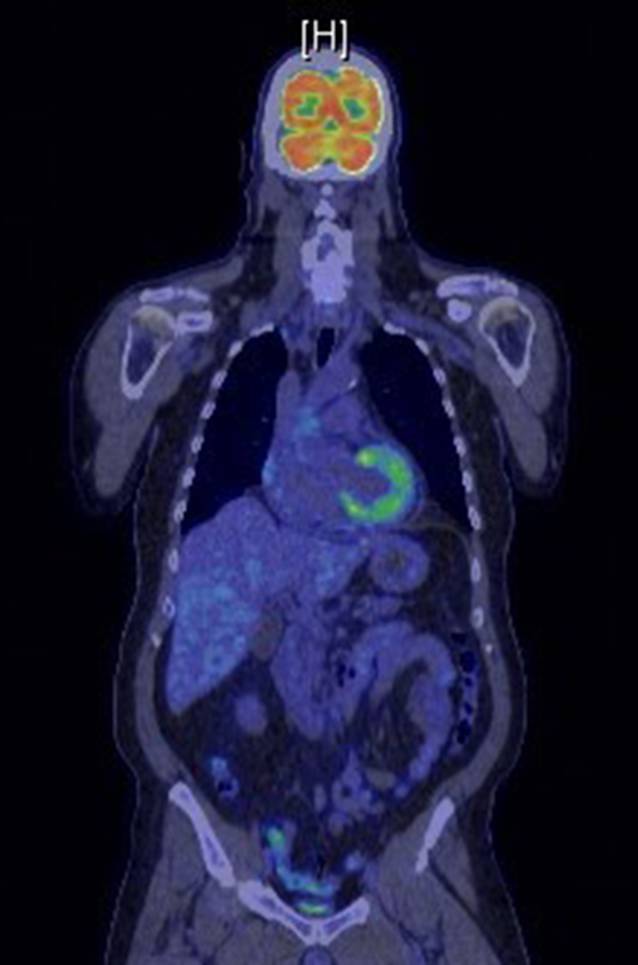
Bei unzureichender Wirksamkeit von Ibuprofen, Colchicin und Prednisolon erfolgte eine Umstellung auf den IL-1-Rezeptorantagonist Anakinra 100 mg s.c. täglich. Hierunter zeigte sich ein promptes klinisches, laborchemisches und sonographisches Therapieansprechen. Bei anhaltender Symptomfreiheit wurde Anakinra nach 6 Monaten abgesetzt. Innerhalb von Tagen nach dem Absetzen bekam der Patient ein erneutes Rezidiv, das ebenfalls erfolgreich mit Anakinra behandelt werden konnte.
